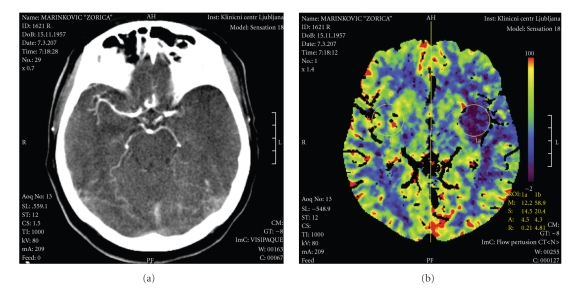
(a) Contrast-enhanced cranial CT with discontinuation of left MCA. (b) CT perfusion test revealed increased time to peak in the velocity.
